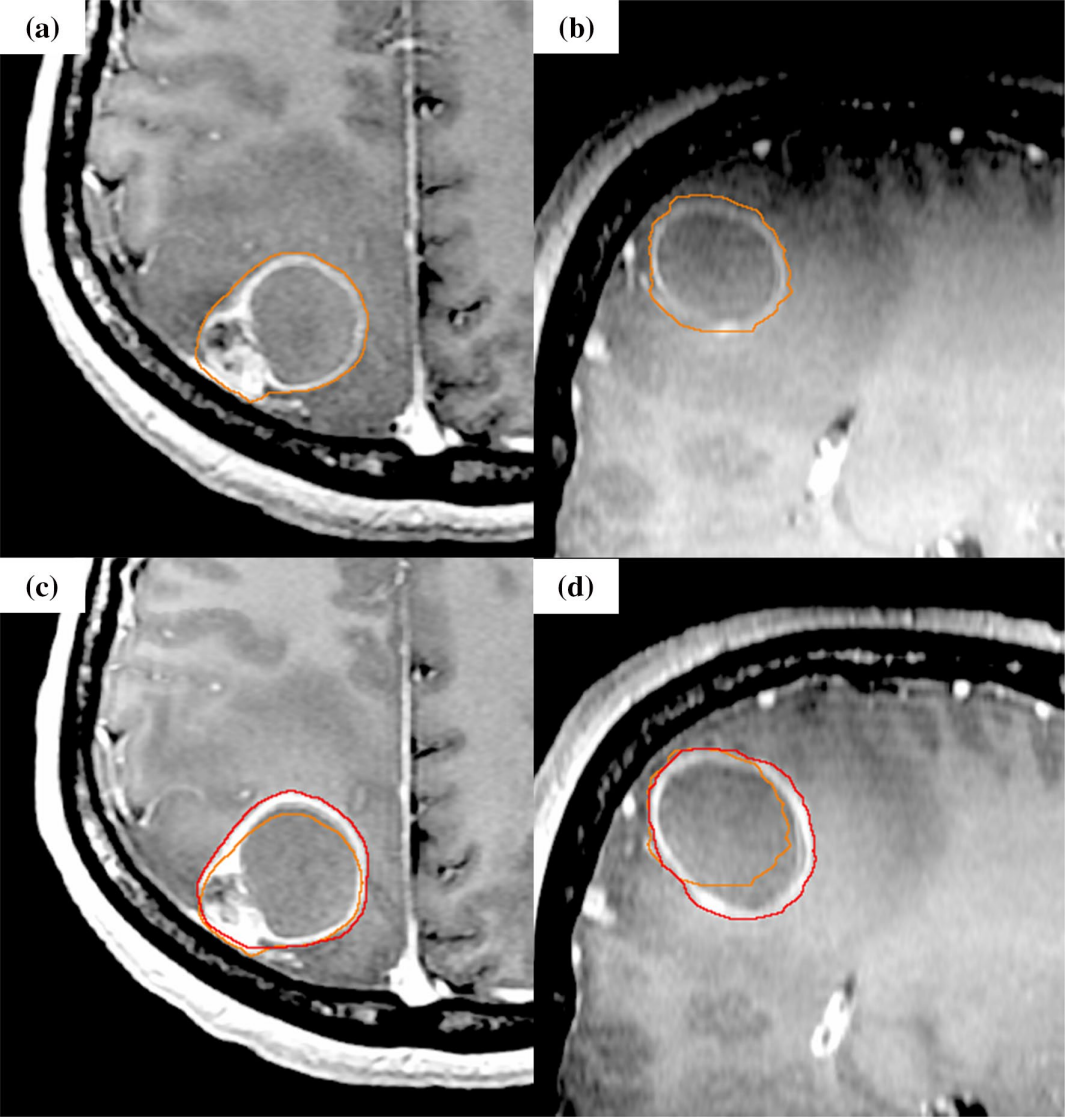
The case of tumor enlargement during stereotactic radiotherapy. The case was brain metastasis of which primary tumor was skin melanoma. Orange line shows the gross tumor volume (GTV) of the initial plan, and red line shows GTV at the modification. The enlargement of cystic component was observed. a, b Axial, Sagittal: T1-weighted magnetic resonance imaging (MRI) with gadolinium (Gd) of the initial plan. c, d Axial, Sagittal: T1-weighted MRI with Gd before fourth treatment for the modification (the 6th day of SRT start)
